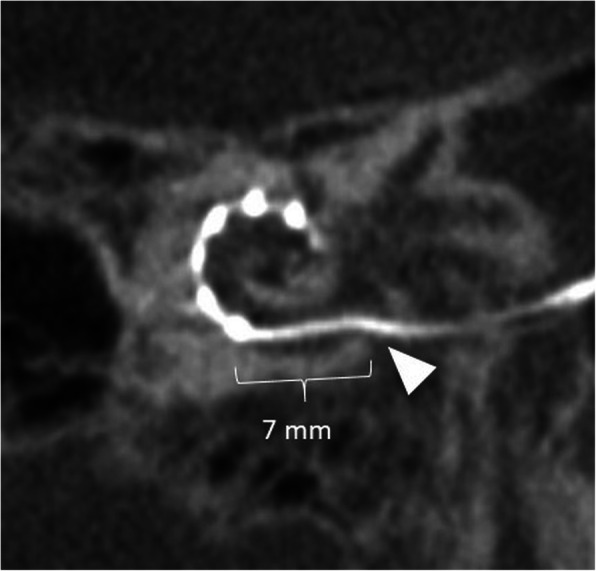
A 11-year-old male patient, with overinserted electrode. CBCT paraaxial maximum intensity projection image shows the most basal electrode contact 7 mm from the round window (arrowhead)
